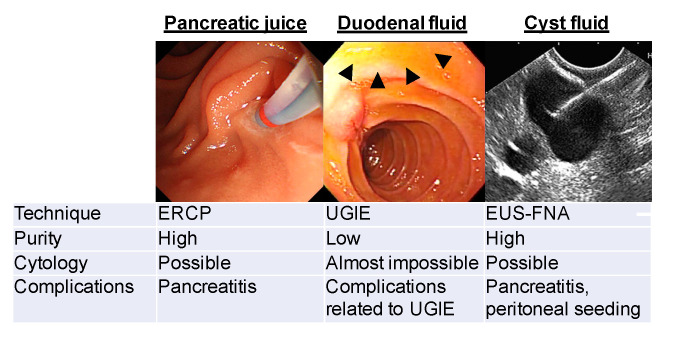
Representative methods to collect pancreatic juice. ERCP: endoscopic retrograde cholangiopancreatography; GIE: gastrointestinal endoscopy; EUS-ENA: endoscopic ultrasonography-guided fine needle aspiration; PJ: pancreatic juice. Black triangle: accumulated duodenal fluid. Scale bar: 1 cm.
